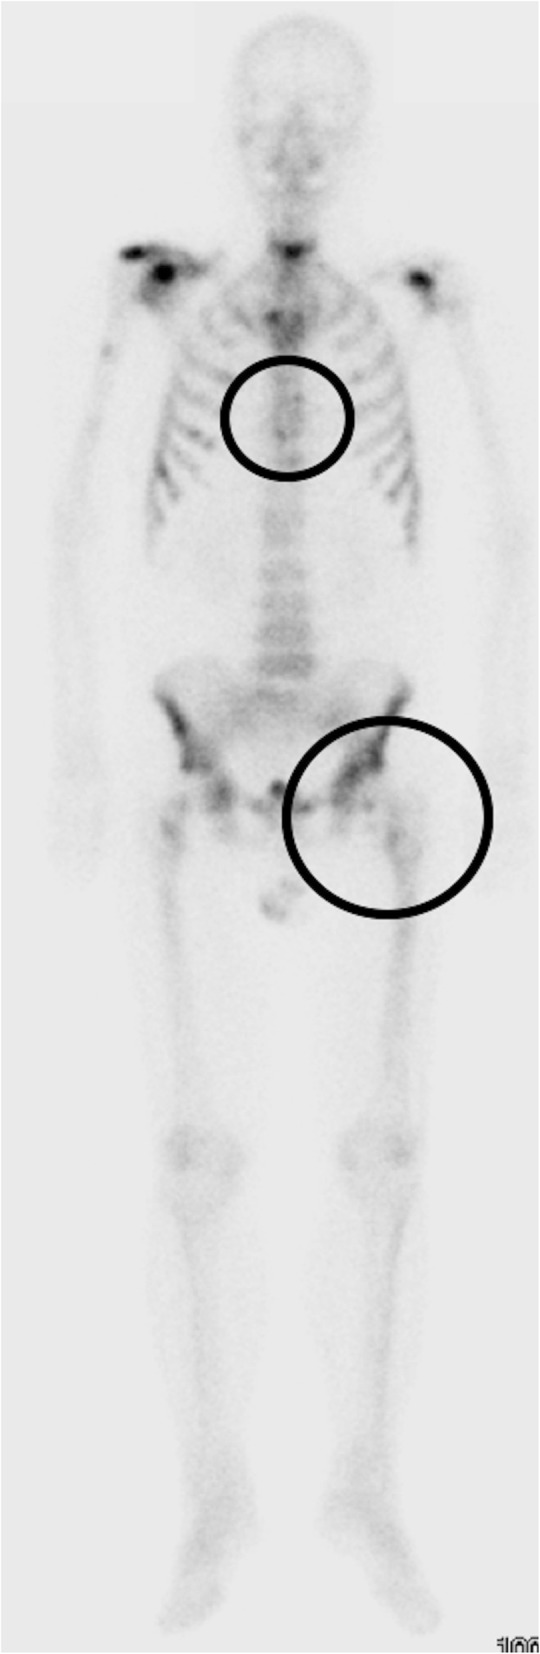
Bone scintigraphy shows multiple bone metastases, especially in the thoracic spine (Th7) and the left femur, where local therapy was performed
